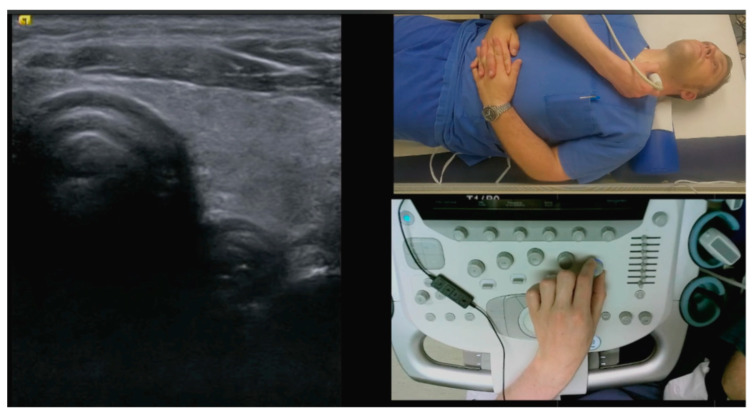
Excerpt from the video guide on how to perform a detailed HANUS examination. The ultrasound transducer- and patient position were displayed in the upper right and the control panel of the ultrasound system in the lower right part of the screen, the corresponding ultrasound image was shown in the left part of the screen. In the audio track of the video, detailed instruction with an explanation of the mapped structures and the handling of the system was given.
